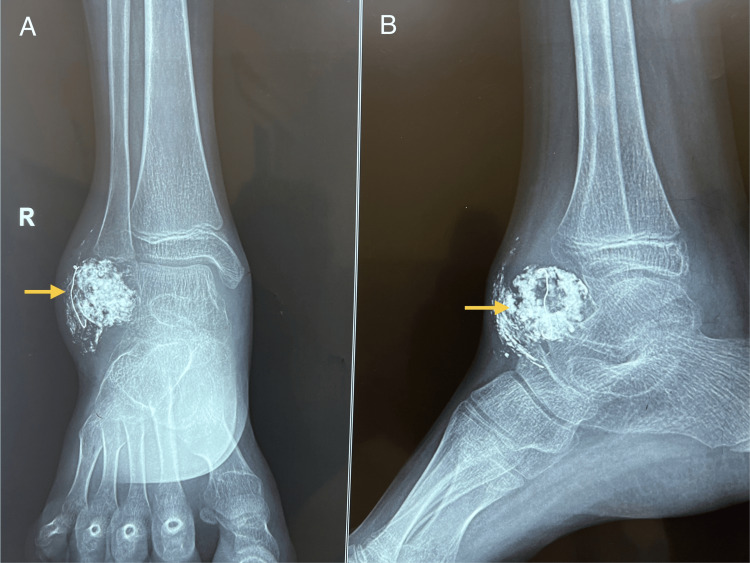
Post-angio-embolization anteroposterior (A) and lateral (B) radiographs of the ankle showing calcification (phlebolith) in the soft tissue tumor with the embolized feeder vessel.
